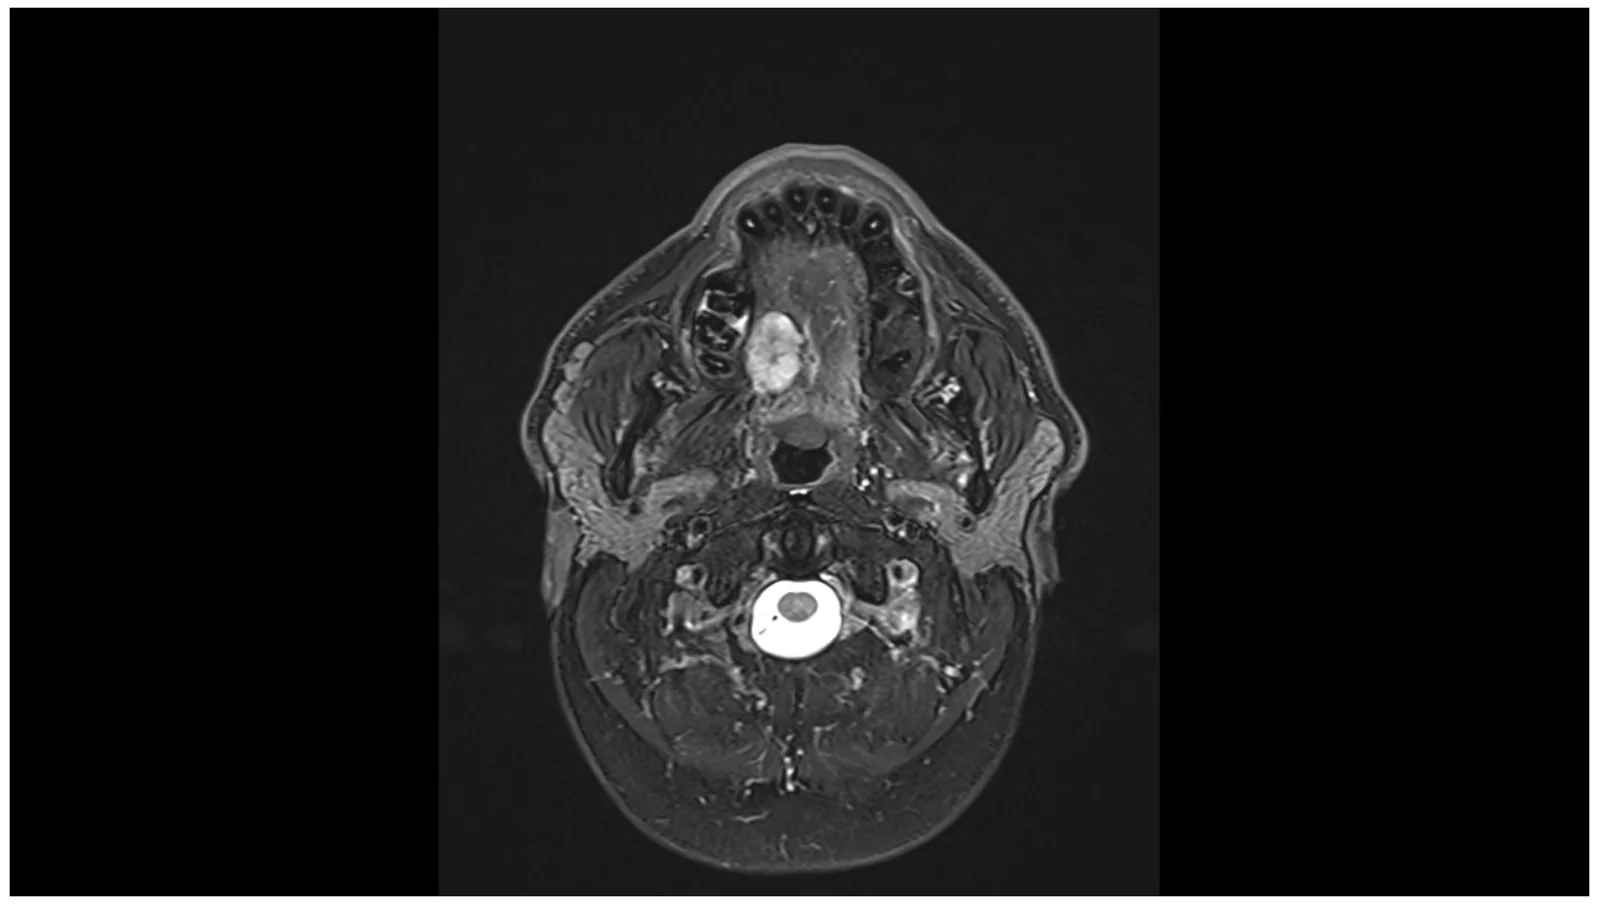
Axial fat-suppressed T2-weighted image demonstrates a well-circumscribed, heterogeneously hyperintense lesion arising from the right posterior palate.
